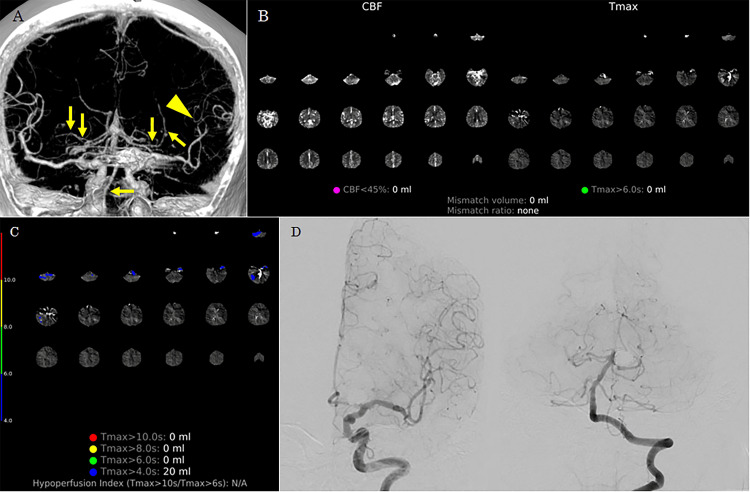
Case 3. (A) CT angiography-like images from RAPID ANGIO suggest possible narrowing of the distal left middle cerebral artery (arrowhead). No vascular narrowing of the posterior circulation was observed (arrows). (B) Automated perfusion maps from RAPID ANGIO show no significant infarct core (cerebral blood flow <45%) or hypoperfused tissue (Tmax >6 seconds). (C) Detailed Tmax maps from RAPID ANGIO suggest mildly delayed perfusion (Tmax >4 seconds) in the posterior circulation. (D) Subsequent left carotid and left vertebral angiography revealed no evidence of cerebral vasospasm.
